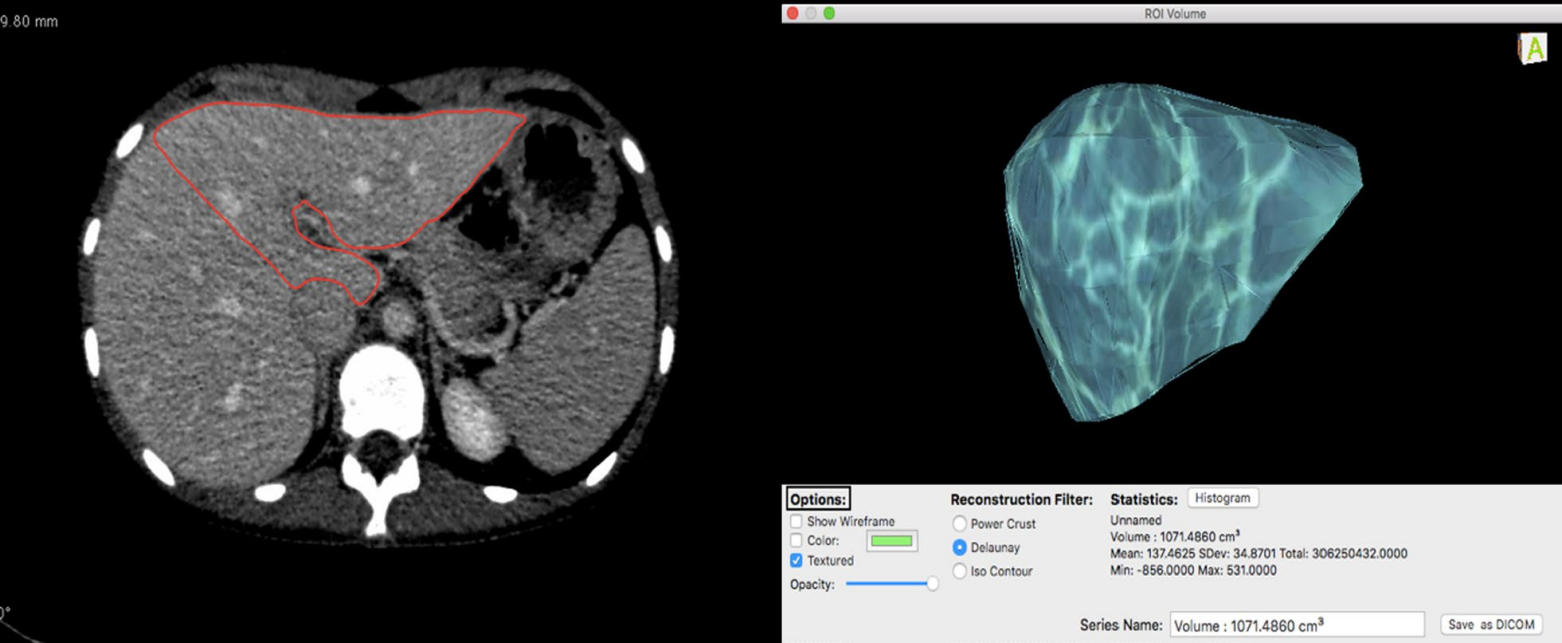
Semi-automated CT volumetry of the liver by OsiriX MD software
